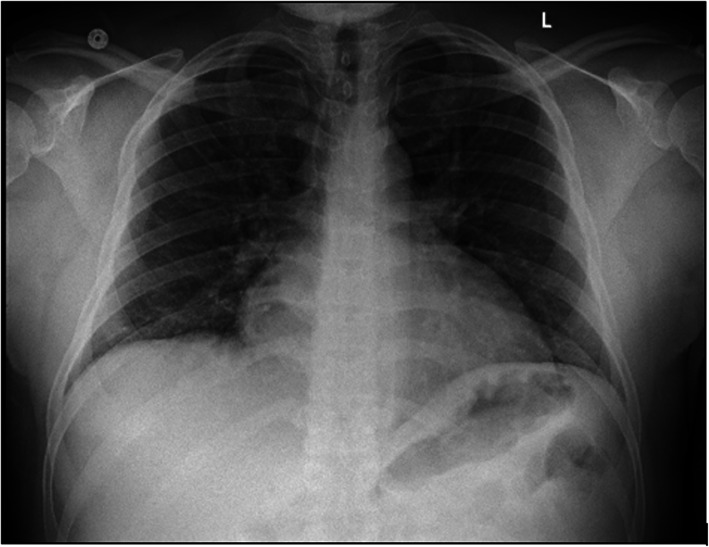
Fig. 1 Chest Xray on admission: No infiltrates noted with COVID19 infection
